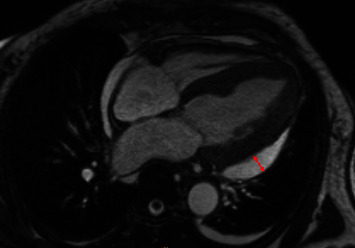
Cardiac MRI 10 days after initial presentation. Axial image captured in mid-diastole shows moderate pericardial effusion (red line) without pericardial or pleural masses.
